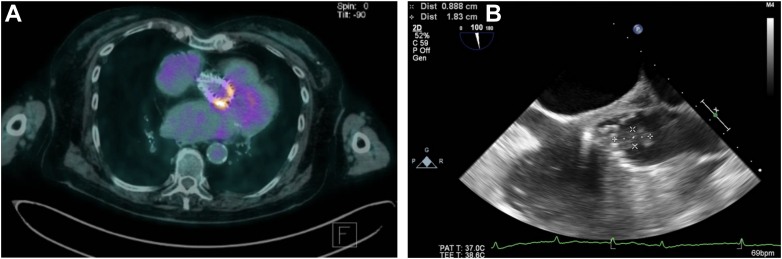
Imaging findings at Initial Presentation (A) PET-CT with enhanced metabolic expression in the proximal and medial area of the transcatheter valve (SUV_max_: 6.4). (B) Transesophageal echocardiogram with thickened leaflets and presence of an irregular, cotton-like mobile mass in the arterial side of the endoprosthesis leaflets, extending into the aortic root, measuring 1.8 cm in length and 0.9 cm in width. PET-CT = positron emission tomography–computed tomography; SUV_max_ = maximum standardized uptake value.
